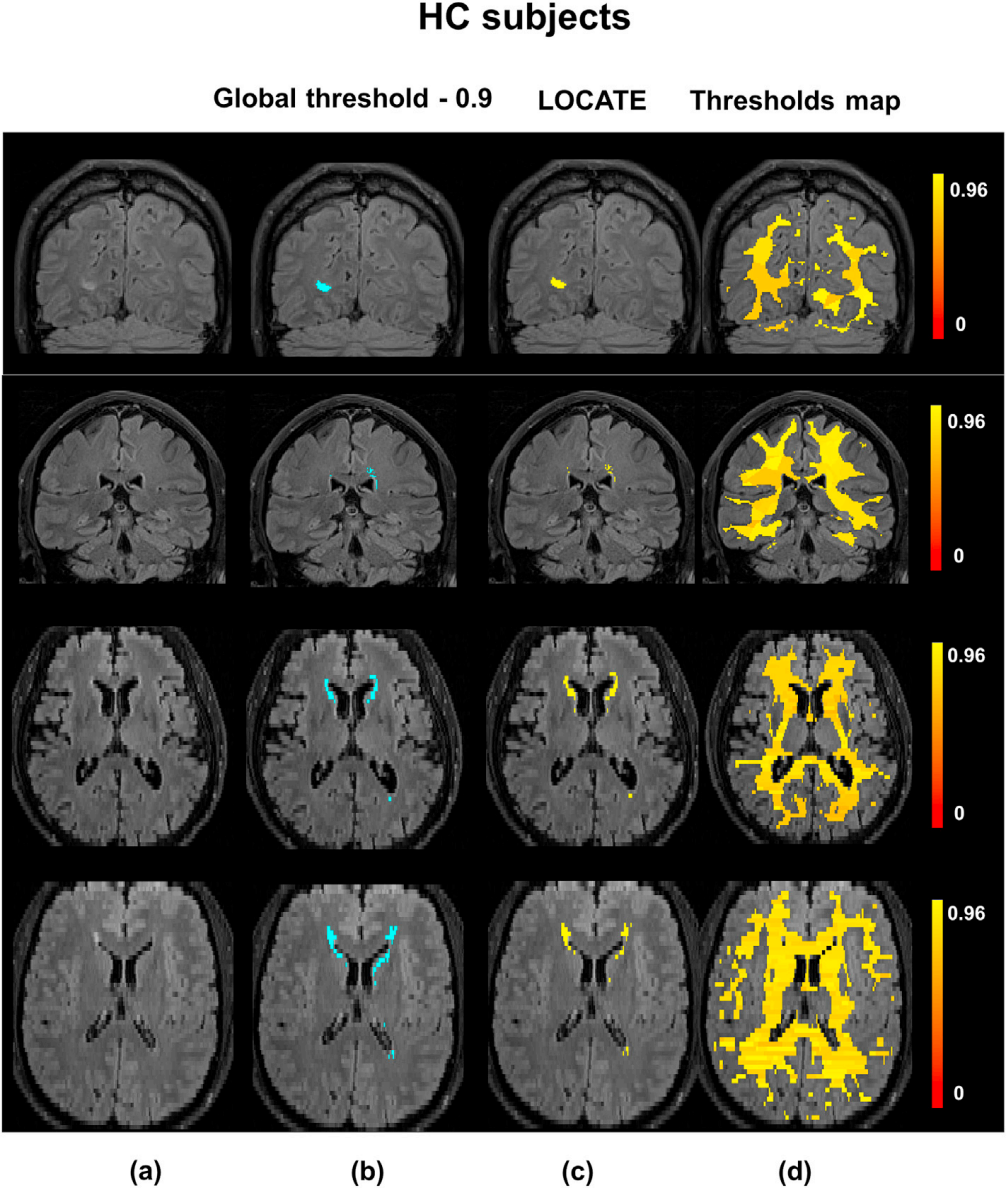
Example results of LOCATE on the HC data. (a) FLAIR image, (b) global thresholding at 0.9 (light blue), (c) LOCATE results (yellow) and (d) threshold maps obtained from LOCATE (red-yellow). Note that the threshold maps shows the heterogeneity in the lesions probabilities in various regions of white matter.
